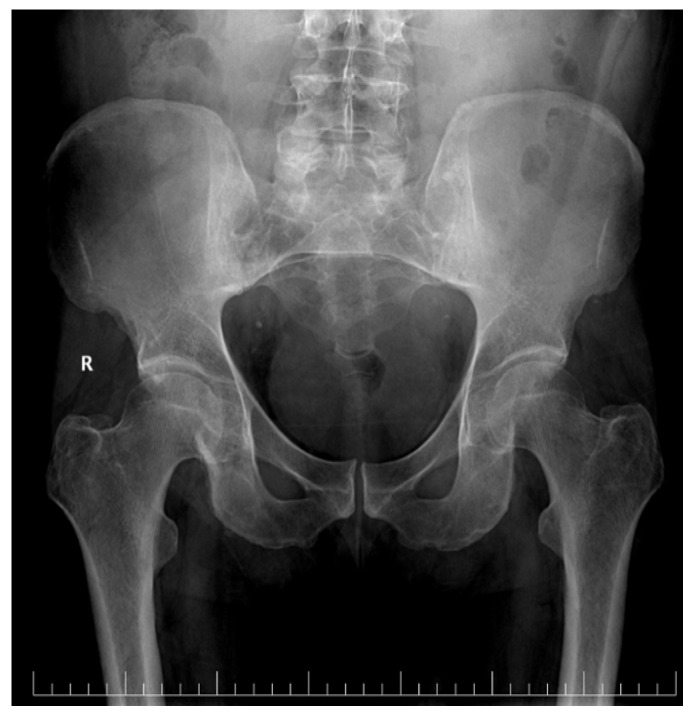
Pelvic X-ray showing narrowing of the right coxofemural joint space.
